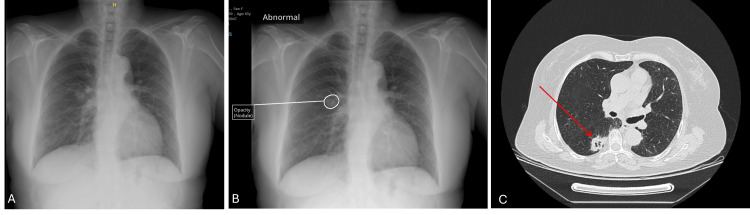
Chest X-ray and CT images of patient 3 A: Chest X-ray image without AI; B: Interpretation of chest X-ray with AI (suspicious nodules marked); C: Computed tomography (CT) image of the thorax (arrows show nodules)
